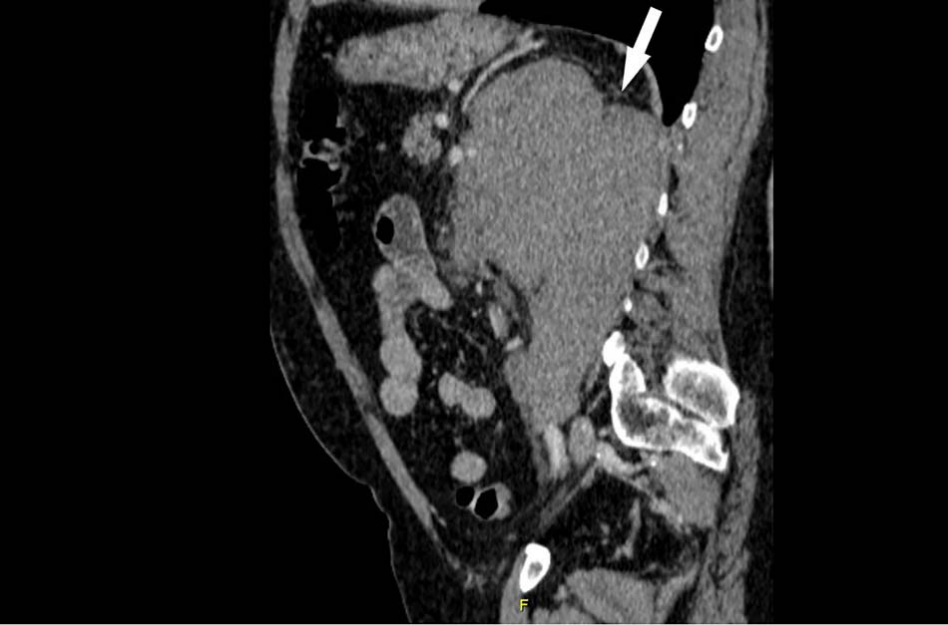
Computed tomography (CT) sagittal section showing predominant bulk of the mass is extrarenal that posteriorly breaches the fascia to involve the diaphragm and psoas muscle. Medially it reaches the intervertebral foramen.
